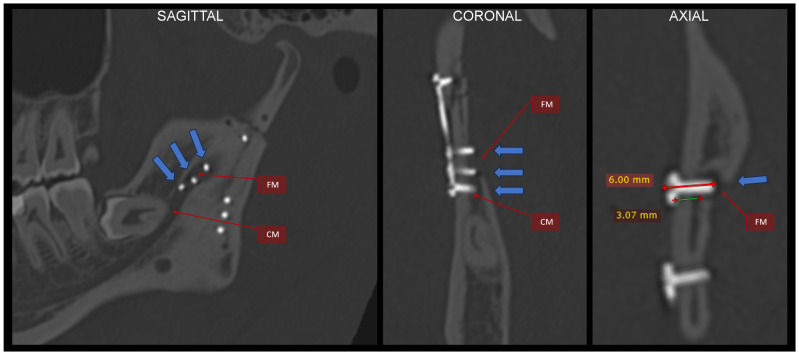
Justification for why short screws should be used. An example of using a plate with two lower divergent arms. In each of these arms in the lower part, there are 3 holes for screws. Three screws located in the middle of the mandibular ramus puncture through the bone and penetrate the mandibular foramen and the initial part of the mandibular canal (see arrows). This position of the implants was revealed by an intraoperative CT scan and was quickly corrected. The blue arrows indicate this medial bone perforation. It is worth noting that in this case, the bone separating the mandibular canal from the outer surface of the ramus is approx. 3 mm. If one adds the thickness of the plate (1.0–1.3 mm), then the screws that are 4 mm in length will not reach the mandibular canal. The radiological multiplanar reconstructions were performed using RadiAnt (www.radiantviewer.com/en; access date: 30 November 2024). Abbreviations: FM—foramen mandibulae; CM—canalis mandibulae.
